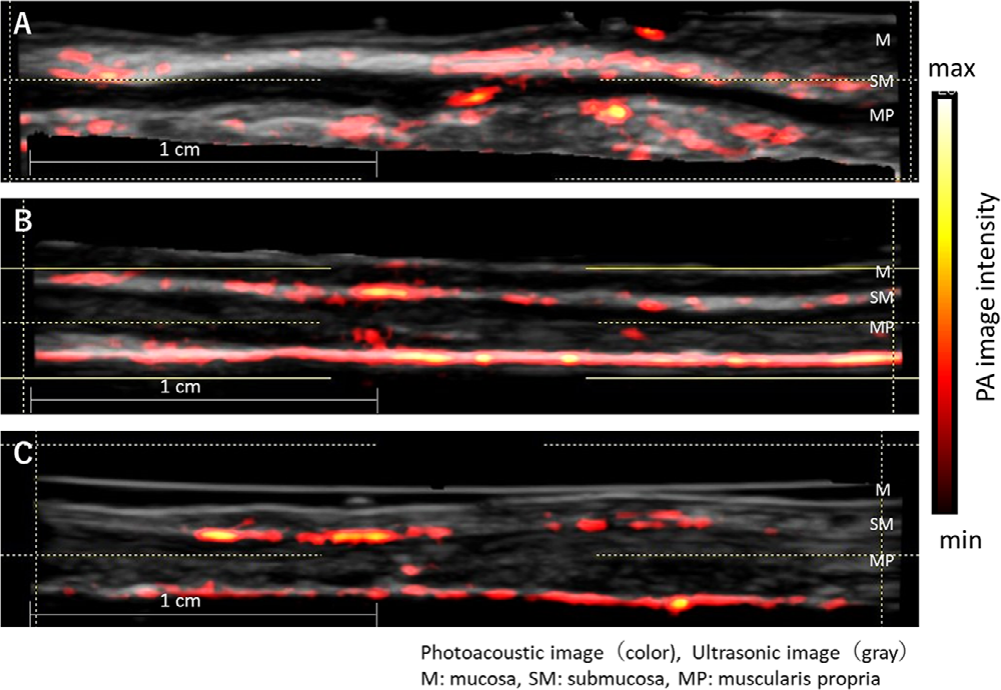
Photoacoustic (PA) image of normal tissue. PA image is superimposed on gray‐scaled B‐mode ultrasound (US) image. The color bar indicates the photoacoustic image intensity. (A) Esophagus, (B) stomach, and (C) rectum
